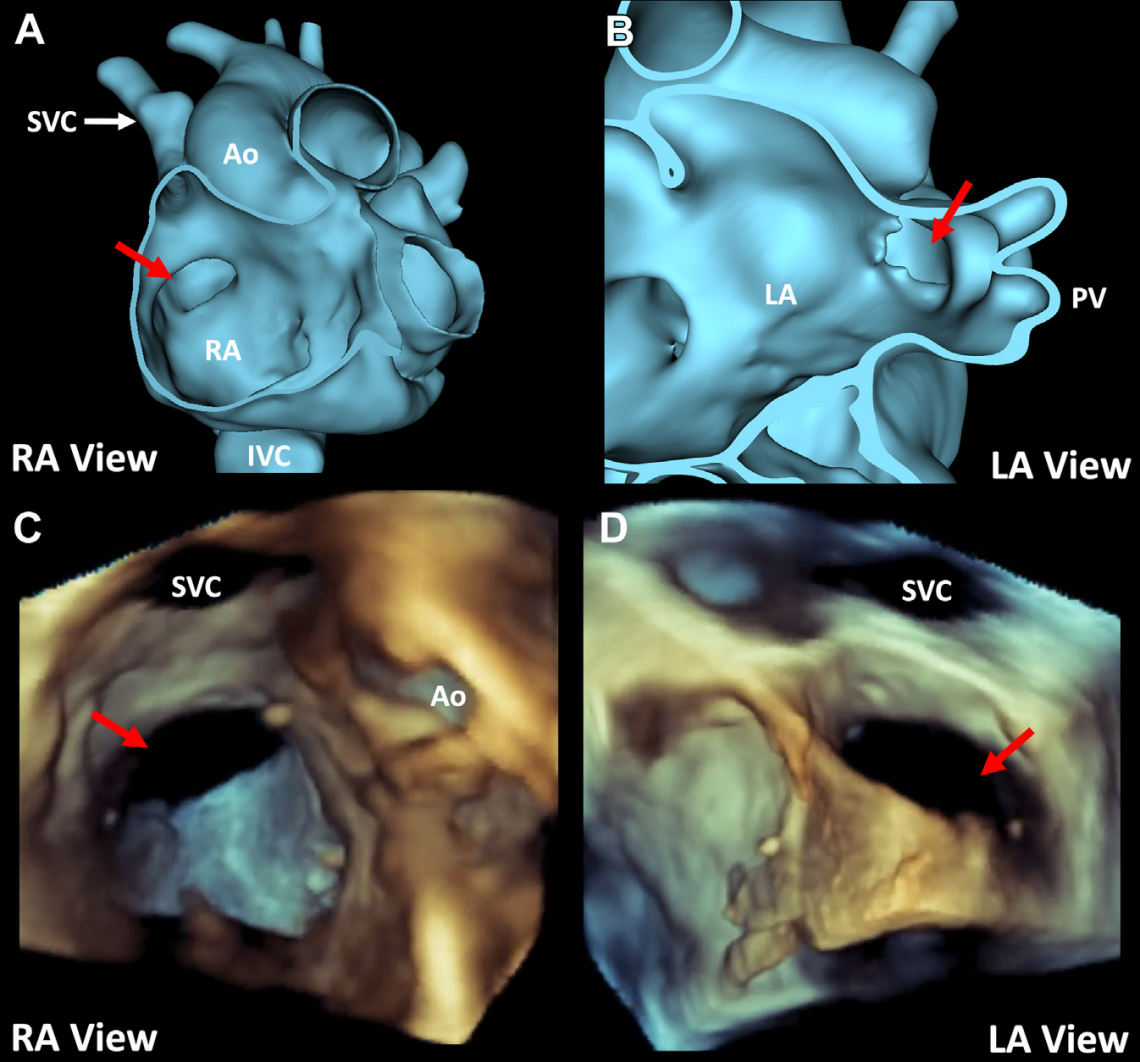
Comparison of Modeling and Procedural 3D TEE (A) Segmentation cut into from lateral right perspective. (B) Left lateral view of site of patch dehiscence (red arrow) (C) 3D transesophageal echocardiogram (TEE) from right atrial (RA) perspective. (D) 3D TEE from left atrial view (LA). SVC = superior vena cava; Ao = aorta.
